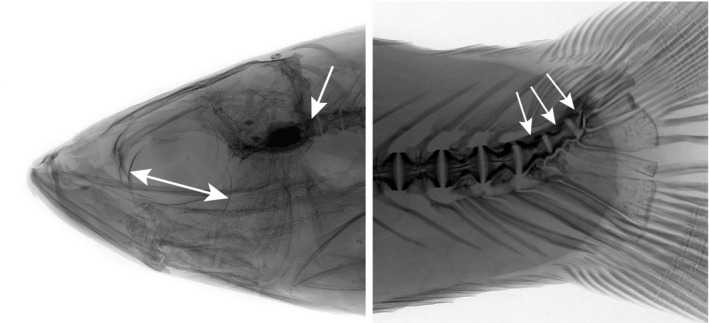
Radiograph images of head and caudal skeleton of *C. albula*, describing measurement of eye diameter, position of the Atlas vertebra, and the three last vertebrae (herein included in total counts)
